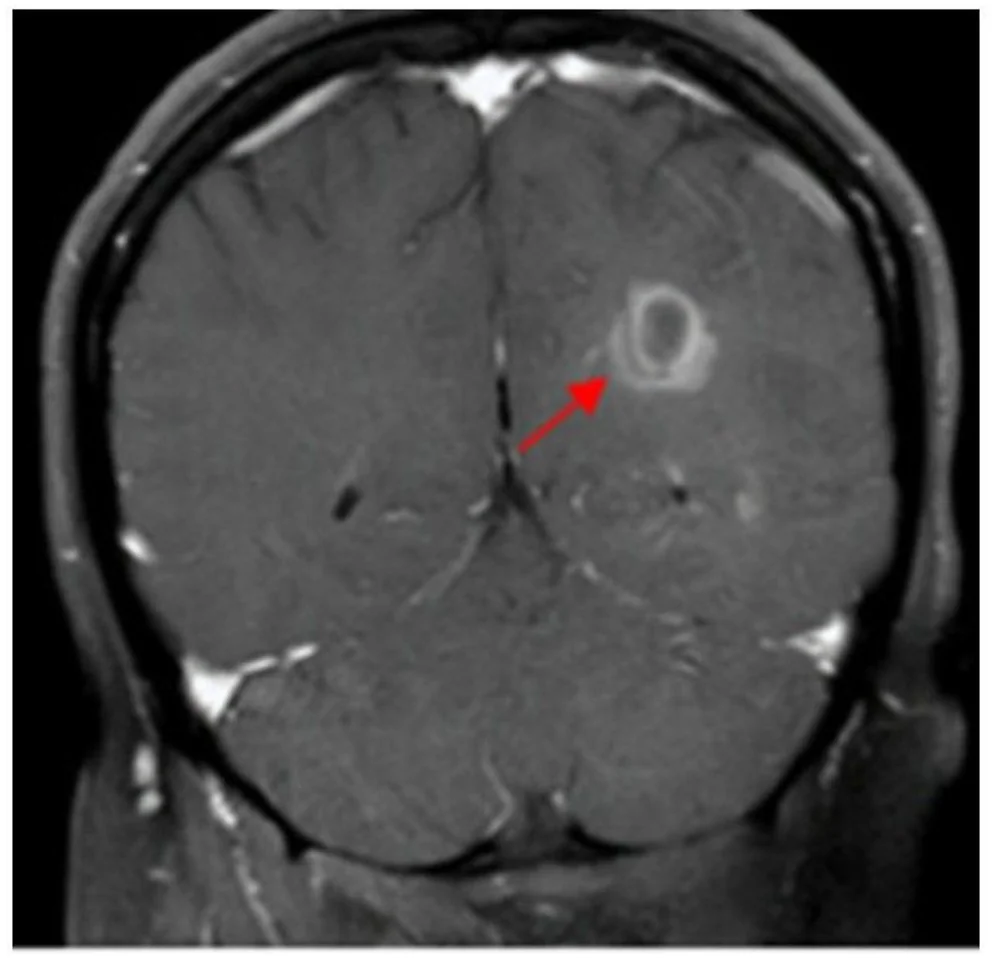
Post‐contrast MRI Brain coronal view obtained day 18 after symptom onset, which demonstrates a full ring of layered enhancement, in addition to a separate partial ring of enhancement around the same lesion (arrow).
